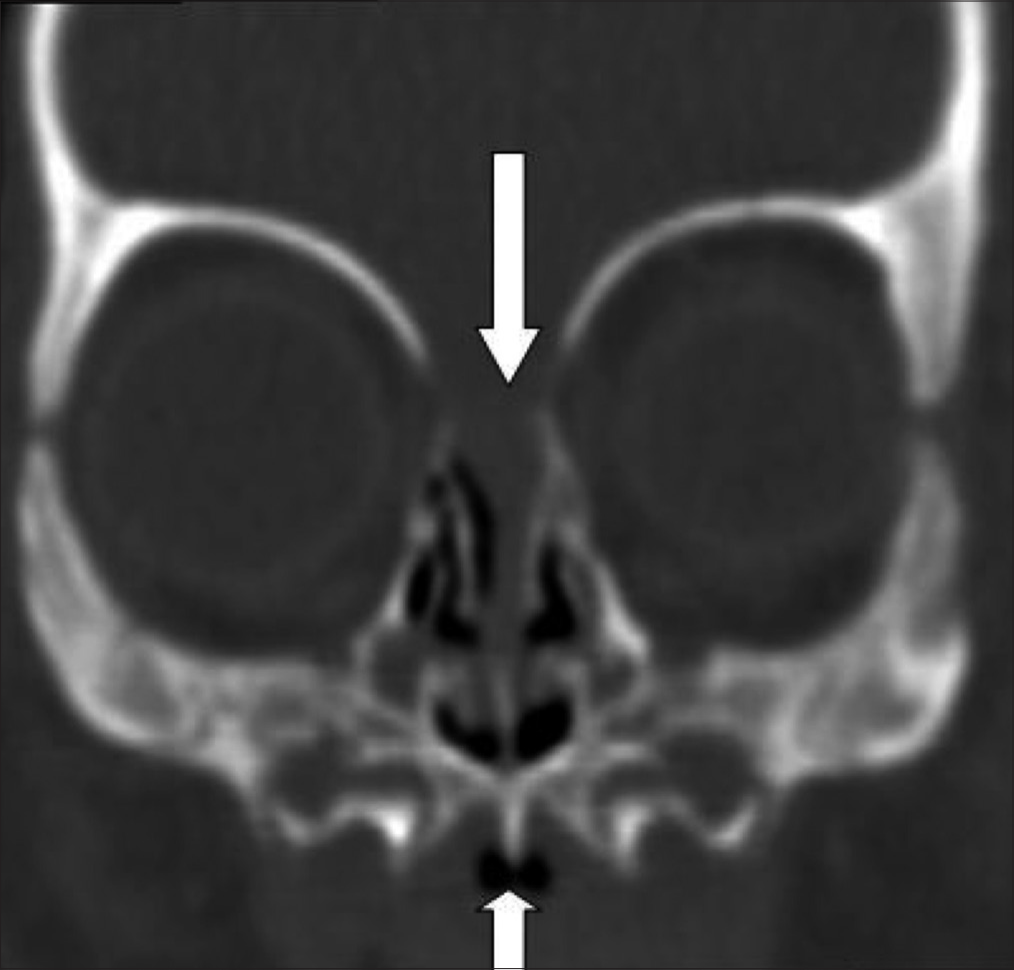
Coronal CT reconstruction shows a prominent inferior palatal ridge (short arrow) and a left nasoethmoid encephalocele (long arrow)
